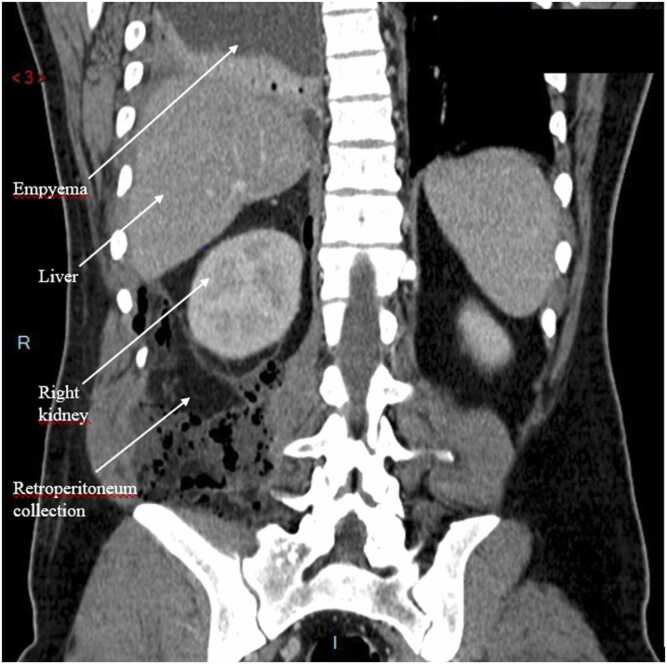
CT scan coronal view of retroperitoneum collection and right-sided empyema.
